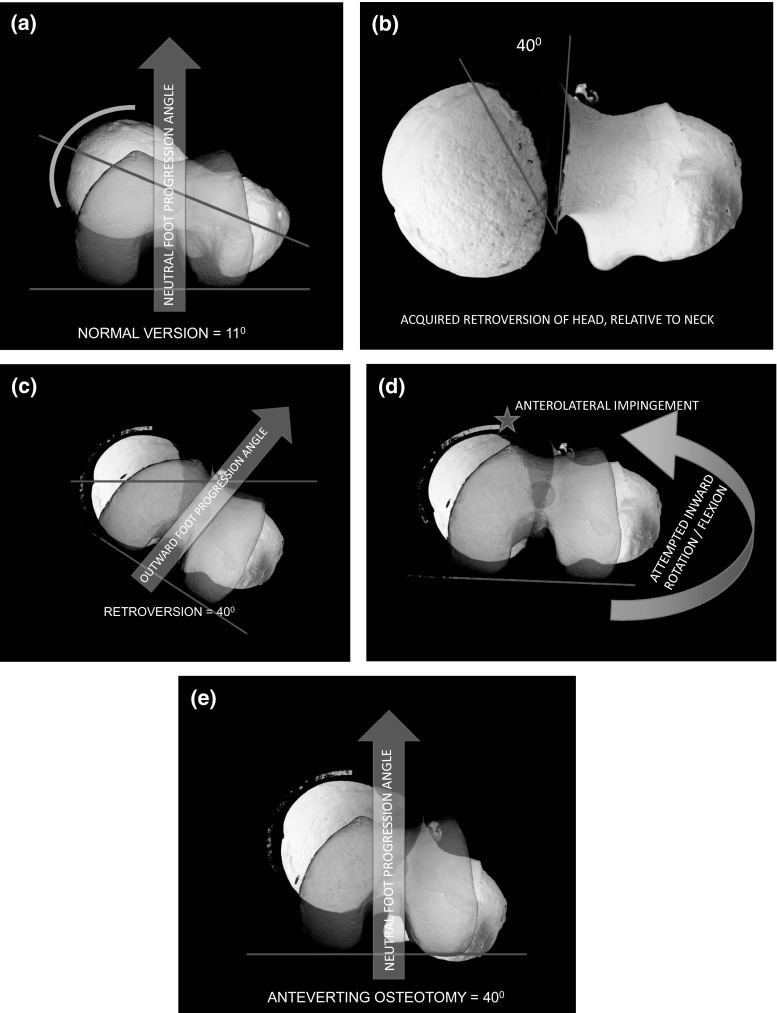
a Normal version of the adolescent or adult femur = 11° and the foot progression angle is neutral. b Acquired retroversion (40° depicted) may be under-recognized because this is interpreted as varus or extension on plain radiographs. c The clinical manifestations include outward foot progression angles noted during gait. d Attempted inward rotation of the hip causes anterolateral impingement, exacerbated by attempted hip flexion. This produces an obligatory outward rotation when walking or sitting. e The rationale for an anteverting osteotomy is shown here, mitigating the impingement in flexion while restoring the neutral foot progressing angle and improving knee kinematics
